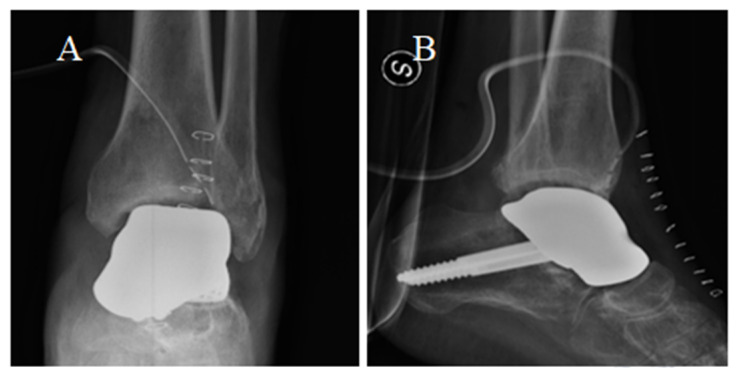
Total talar replacement with subtalar fixation, post-operatory anteroposterior (A) and latero-lateral (B) X-ray.
